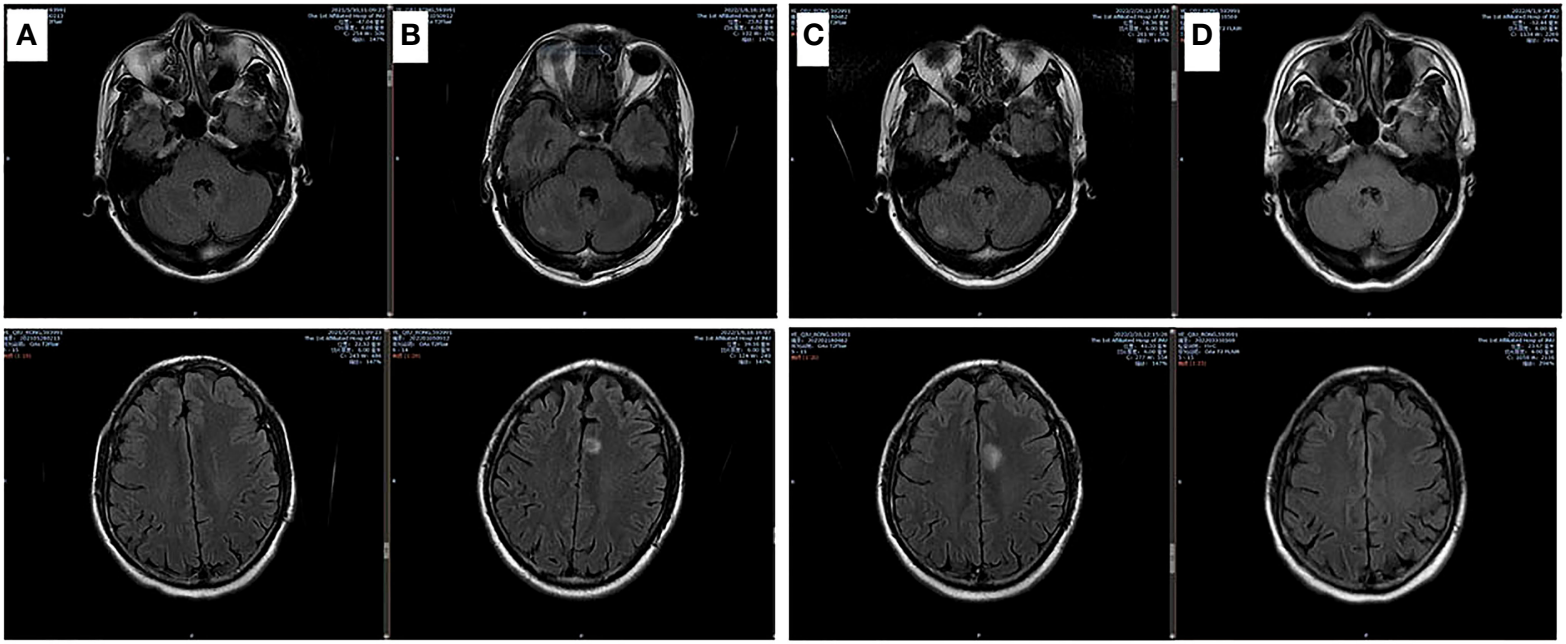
Brain MRI images of involved matastasis images. (A) Base line; (B) After chemotherapy for 5 cycles and lung radiation for 25 cycles; (C) 1 month after newly BMs; (D) 1 month after brain radiation and anlotinib treatment.
